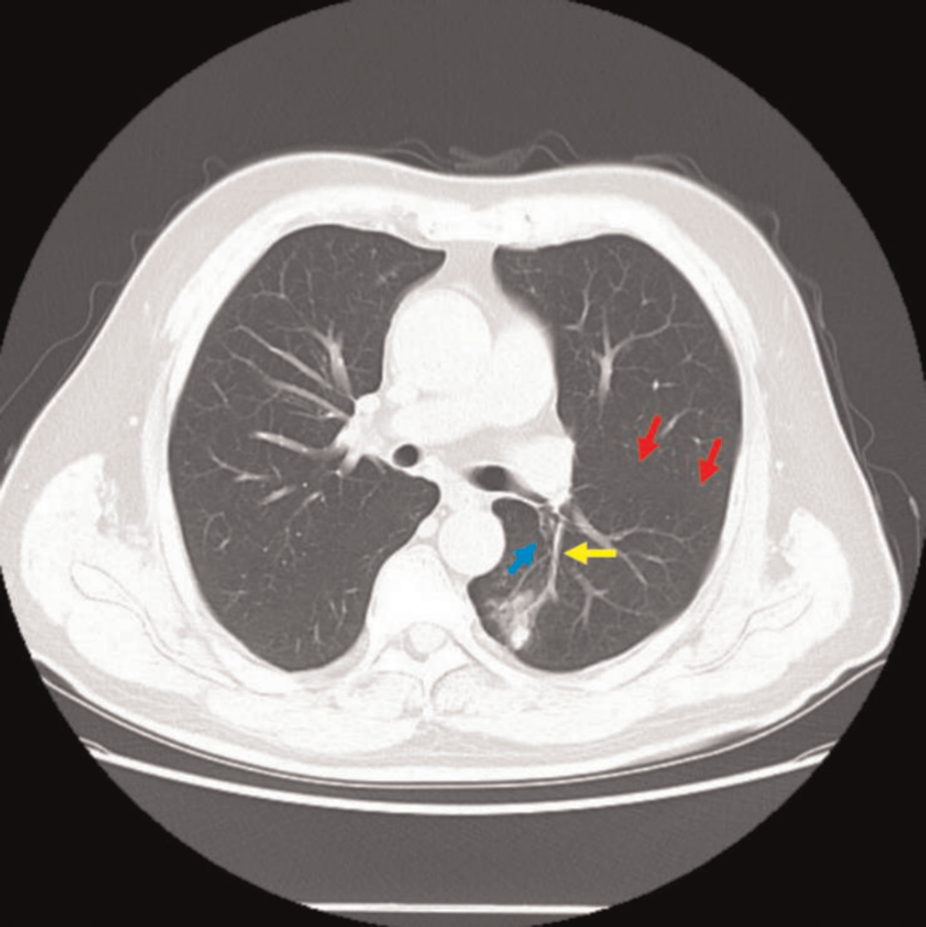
CT imaging of the patient before the surgery. The red arrow refers to the oblique fissure, the yellow arrow refers to the S6 artery, and the blue arrow refers to the S6 bronchus, regarded by radiologists and surgeons.
